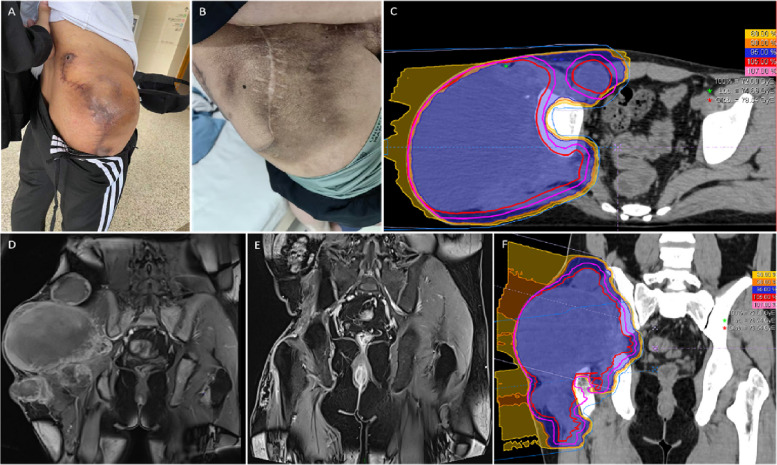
Management of a patient with recurrent CIC‑rearranged sarcoma. This patient presented with local recurrences two months after initial surgery. The patient received CIRT (72 Gy/18 fractions) followed by tumor resection one month later. A, B Clinical photographs of the skin at the lesion site before (A) and 3 years after (B) completion of CIRT and surgery. C, F Axial (C) and coronal (F) views of the CIRT dose distribution. The CTV was prescribed to receive ≥ 95% of the prescribed dose. D, E MRI before (D) and 3 years after (E) CIRT and surgery. In brief. For a detailed clinical summary, treatment parameters, and longitudinal outcome data of this case, please refer to Supplementary Case Report 1
